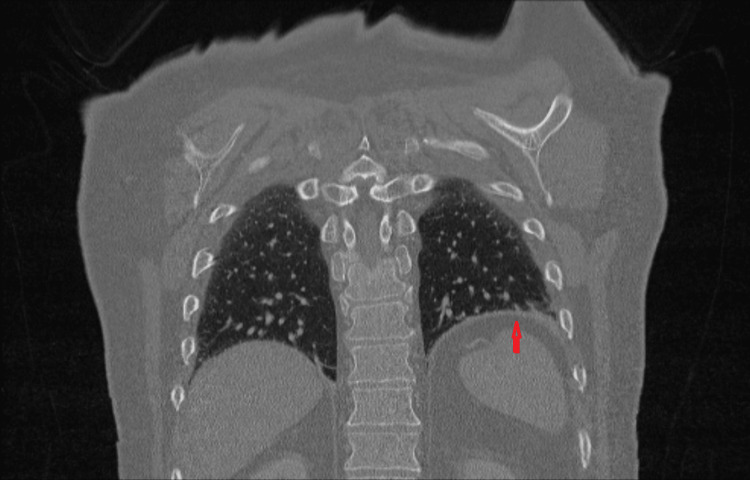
Chest CT demonstrating subtle elevation of the left hemidiaphragm without evidence of pulmonary embolism, interstitial lung disease, or airway obstruction. CT, computed tomography.
